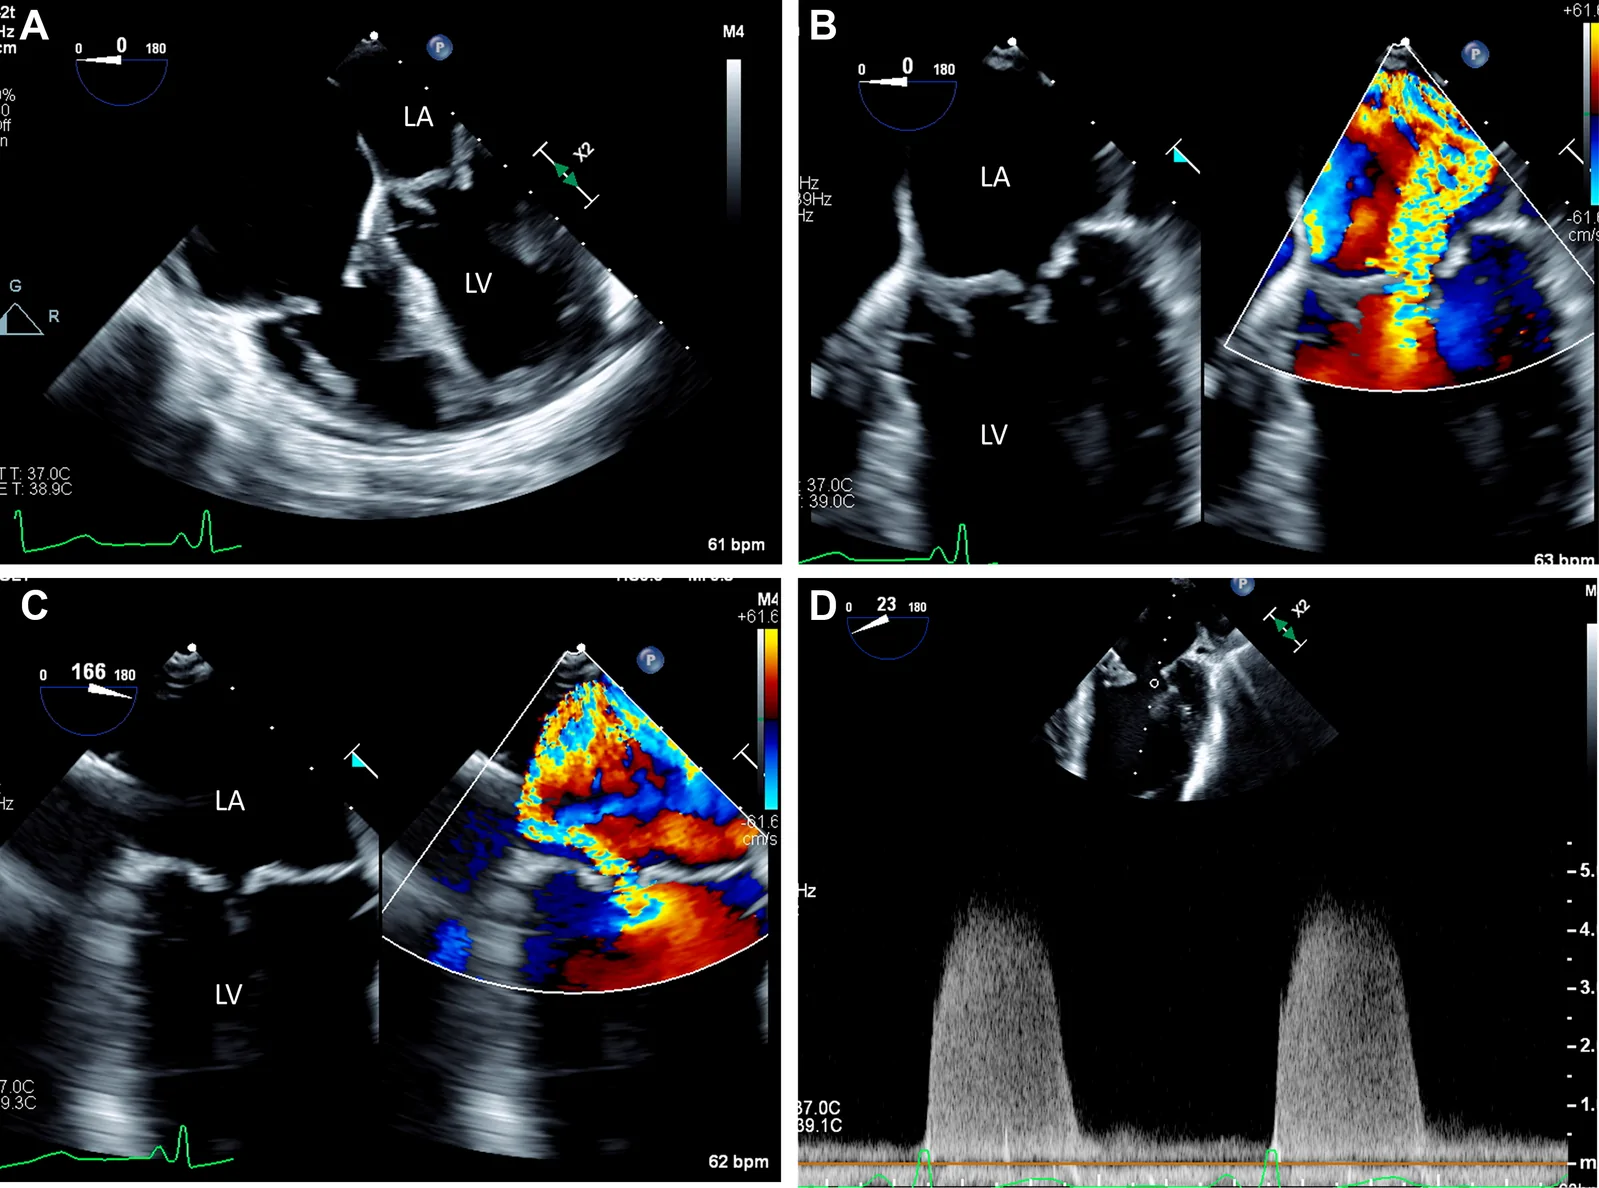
Transesophageal Echocardiography Showing Restricted Mitral Valve With Severe Regurgitation Transesophageal echocardiography midesophageal view showing thickened, mitral valve restricted posterior leaflet and prolapse of the anterior leaflet (A) with resultant severe posteriorly directed mitral regurgitation (B, C). Continuous-wave Doppler with dense jet suggestive of severe MR (D). Abbreviations as in Figure 2.
